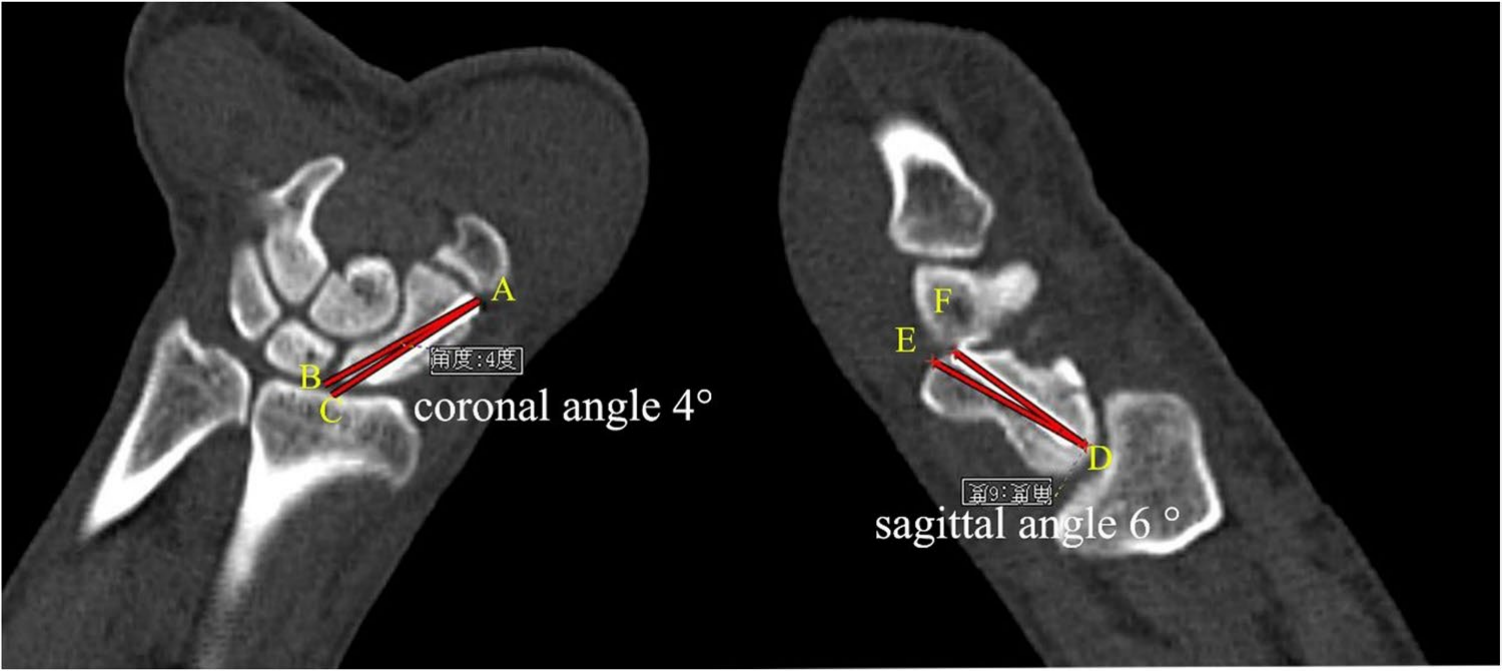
The screw implantation accuracy was evaluated by the angles between the actual position of the screw and the ideal position of the screw (i.e., the central axis of the scaphoid) in post-operative sagittal and coronal CT images
